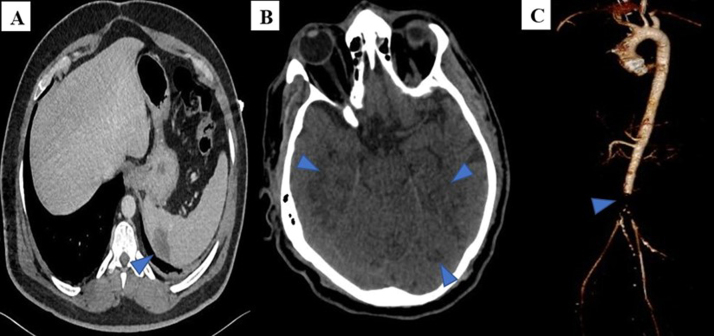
(A) CT abdomen showing wedge-shaped hypodense lesion consistent with splenic infarction, (B) CT brain showing multiple hypodense lesions involving temporal and occipital lobes consistent with infarcts due to showering emboli, (C) CT aortogram depicting occlusion of abdominal aorta bifurcation.
